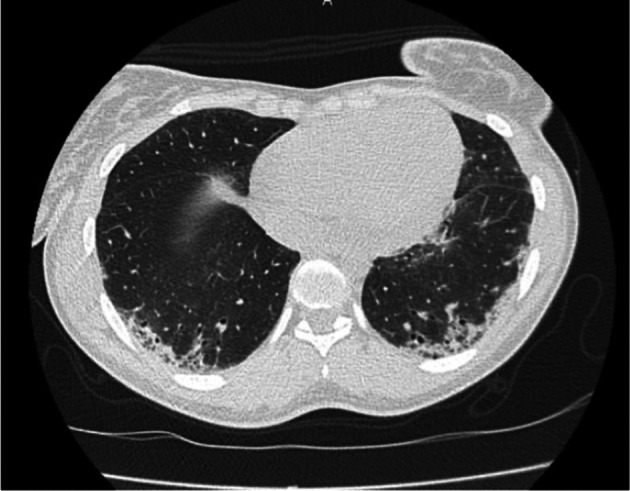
Chest computed tomography showing ground glass opacities suggestive of interstitial pneumonia at pulmonary bases with bronchiectasis.
